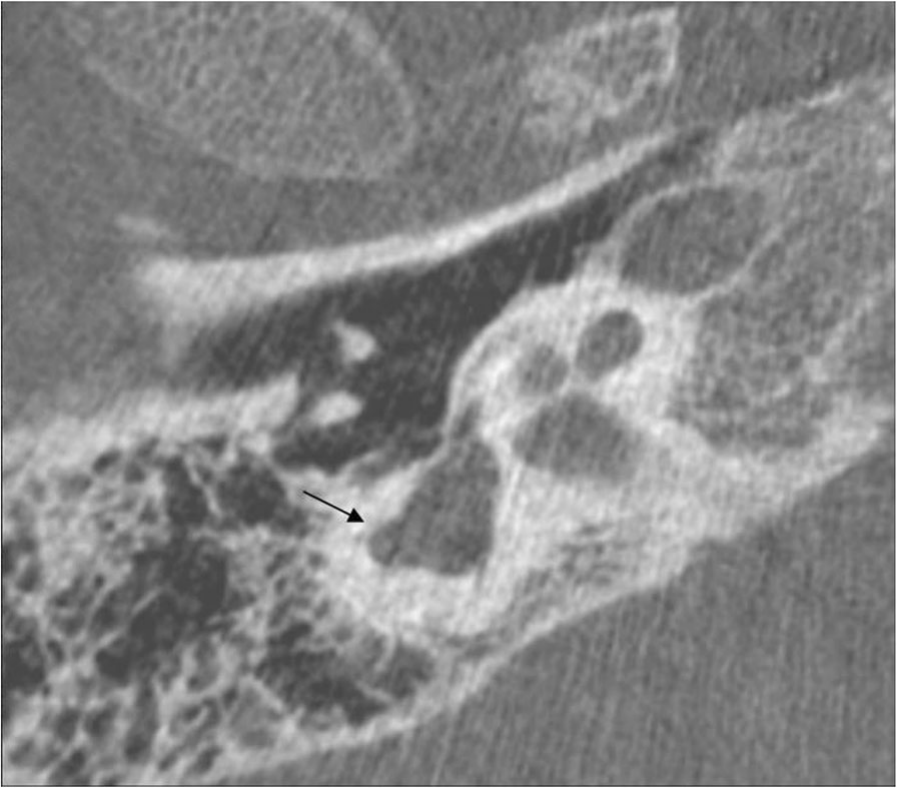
Patient 8’s axial CT image shows the dysplasia of lateral SCC (black arrow) which is only partially present
